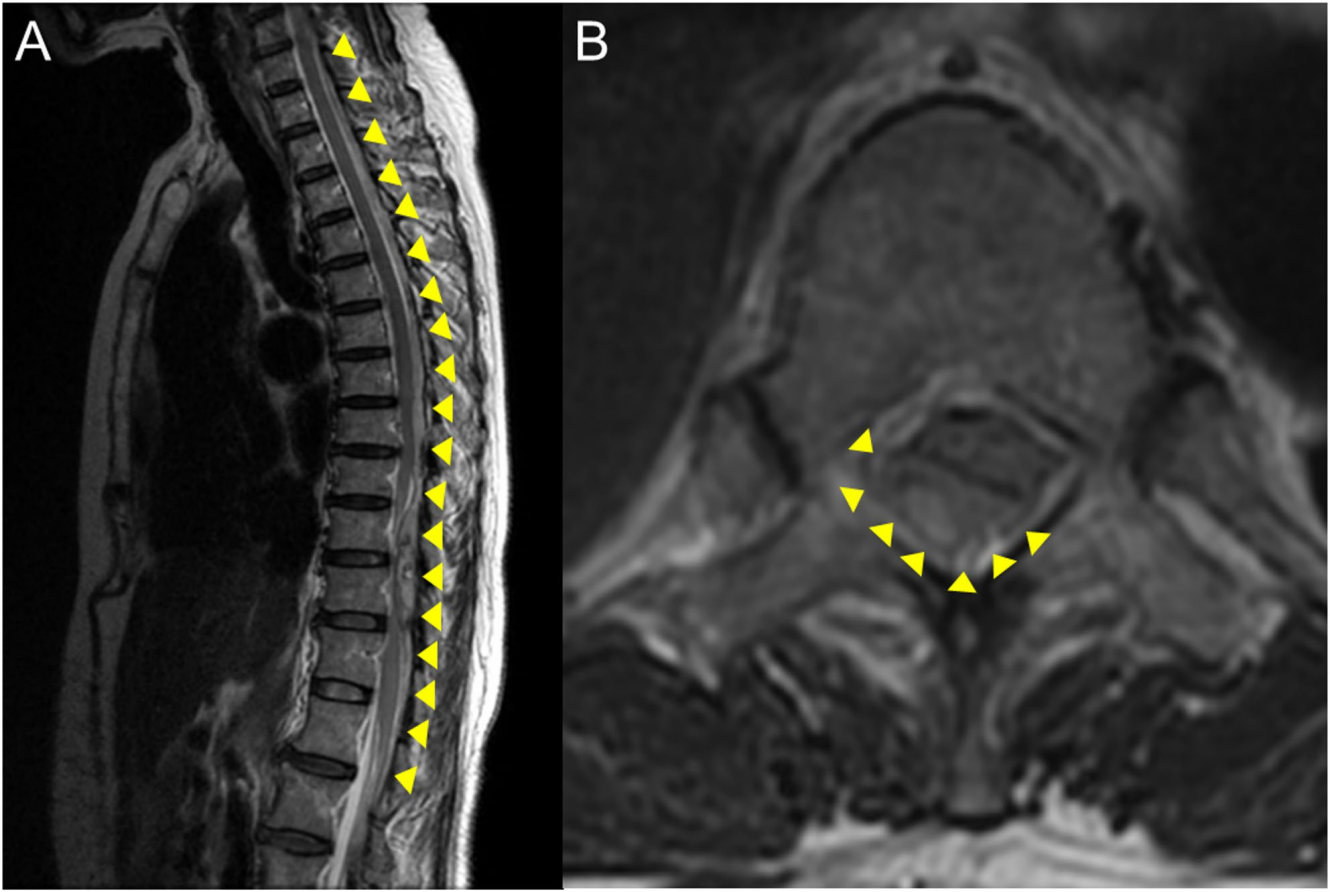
A) T2-weighted sagittal magnetic resonance imaging demonstrating a posterior epidural hematoma extending from C6 to Th12. Spinal cord compression is most evident between Th6 and Th12, with maximal compression at Th10–Th12. B) T2-weighted axial magnetic resonance imaging at Th11 shows that the hematoma occupied approximately 40% of the spinal canal. The hematoma compressed the spinal cord from the right posterior aspect, resulting in slight flattening of the spinal cord. No abnormal signal intensity was detected within the spinal cord
